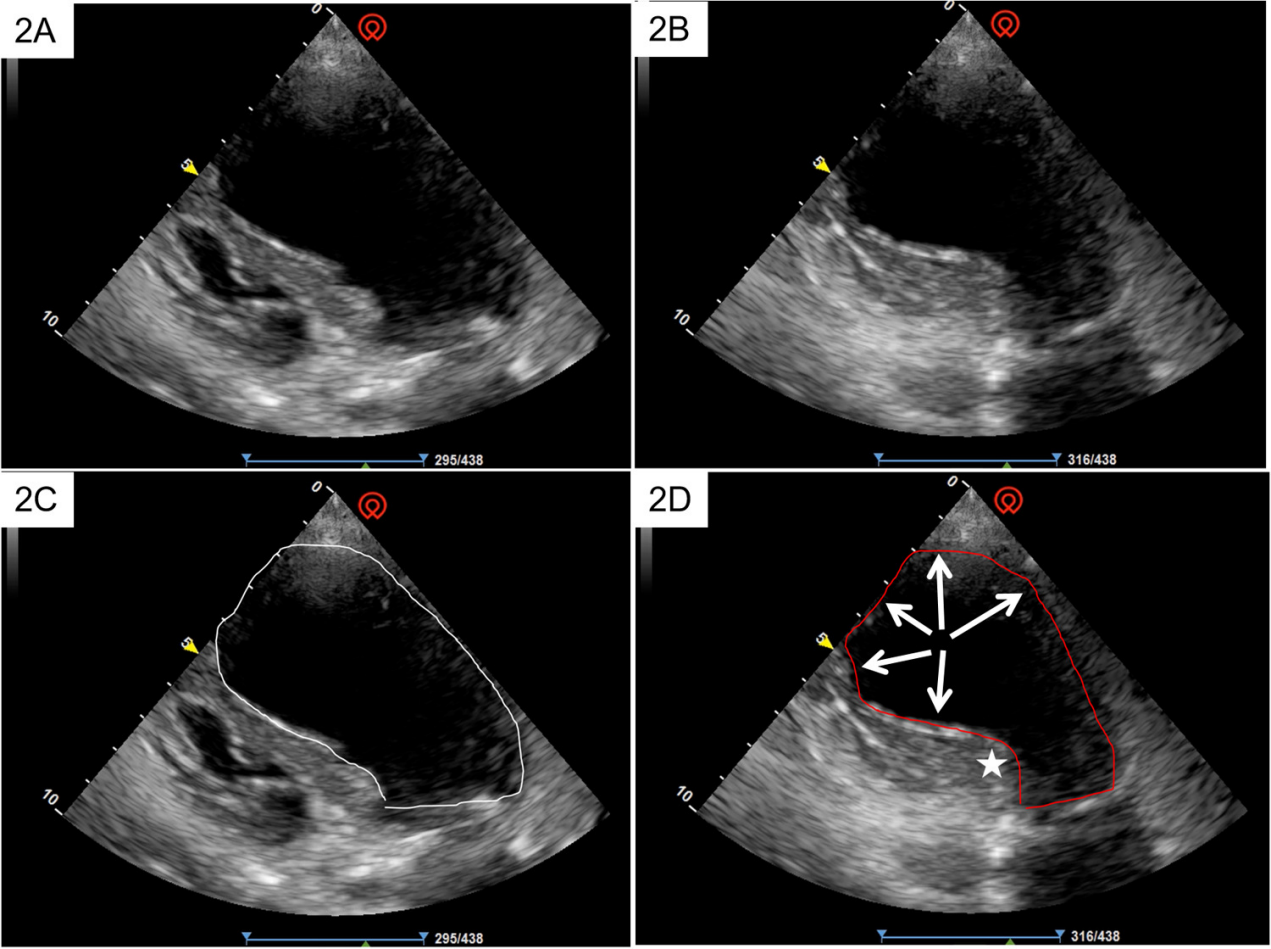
The echocardiographic manifestations of the long axis of the heart at the end of ventricular diastole (A,C), and at the end of ventricular systole (B,D). Comparative analysis demonstrated that only the basal portion of the ventricle exhibited contraction (indicated by the asterisk), whereas the apex scarcely contracted (indicated by the arrow).
